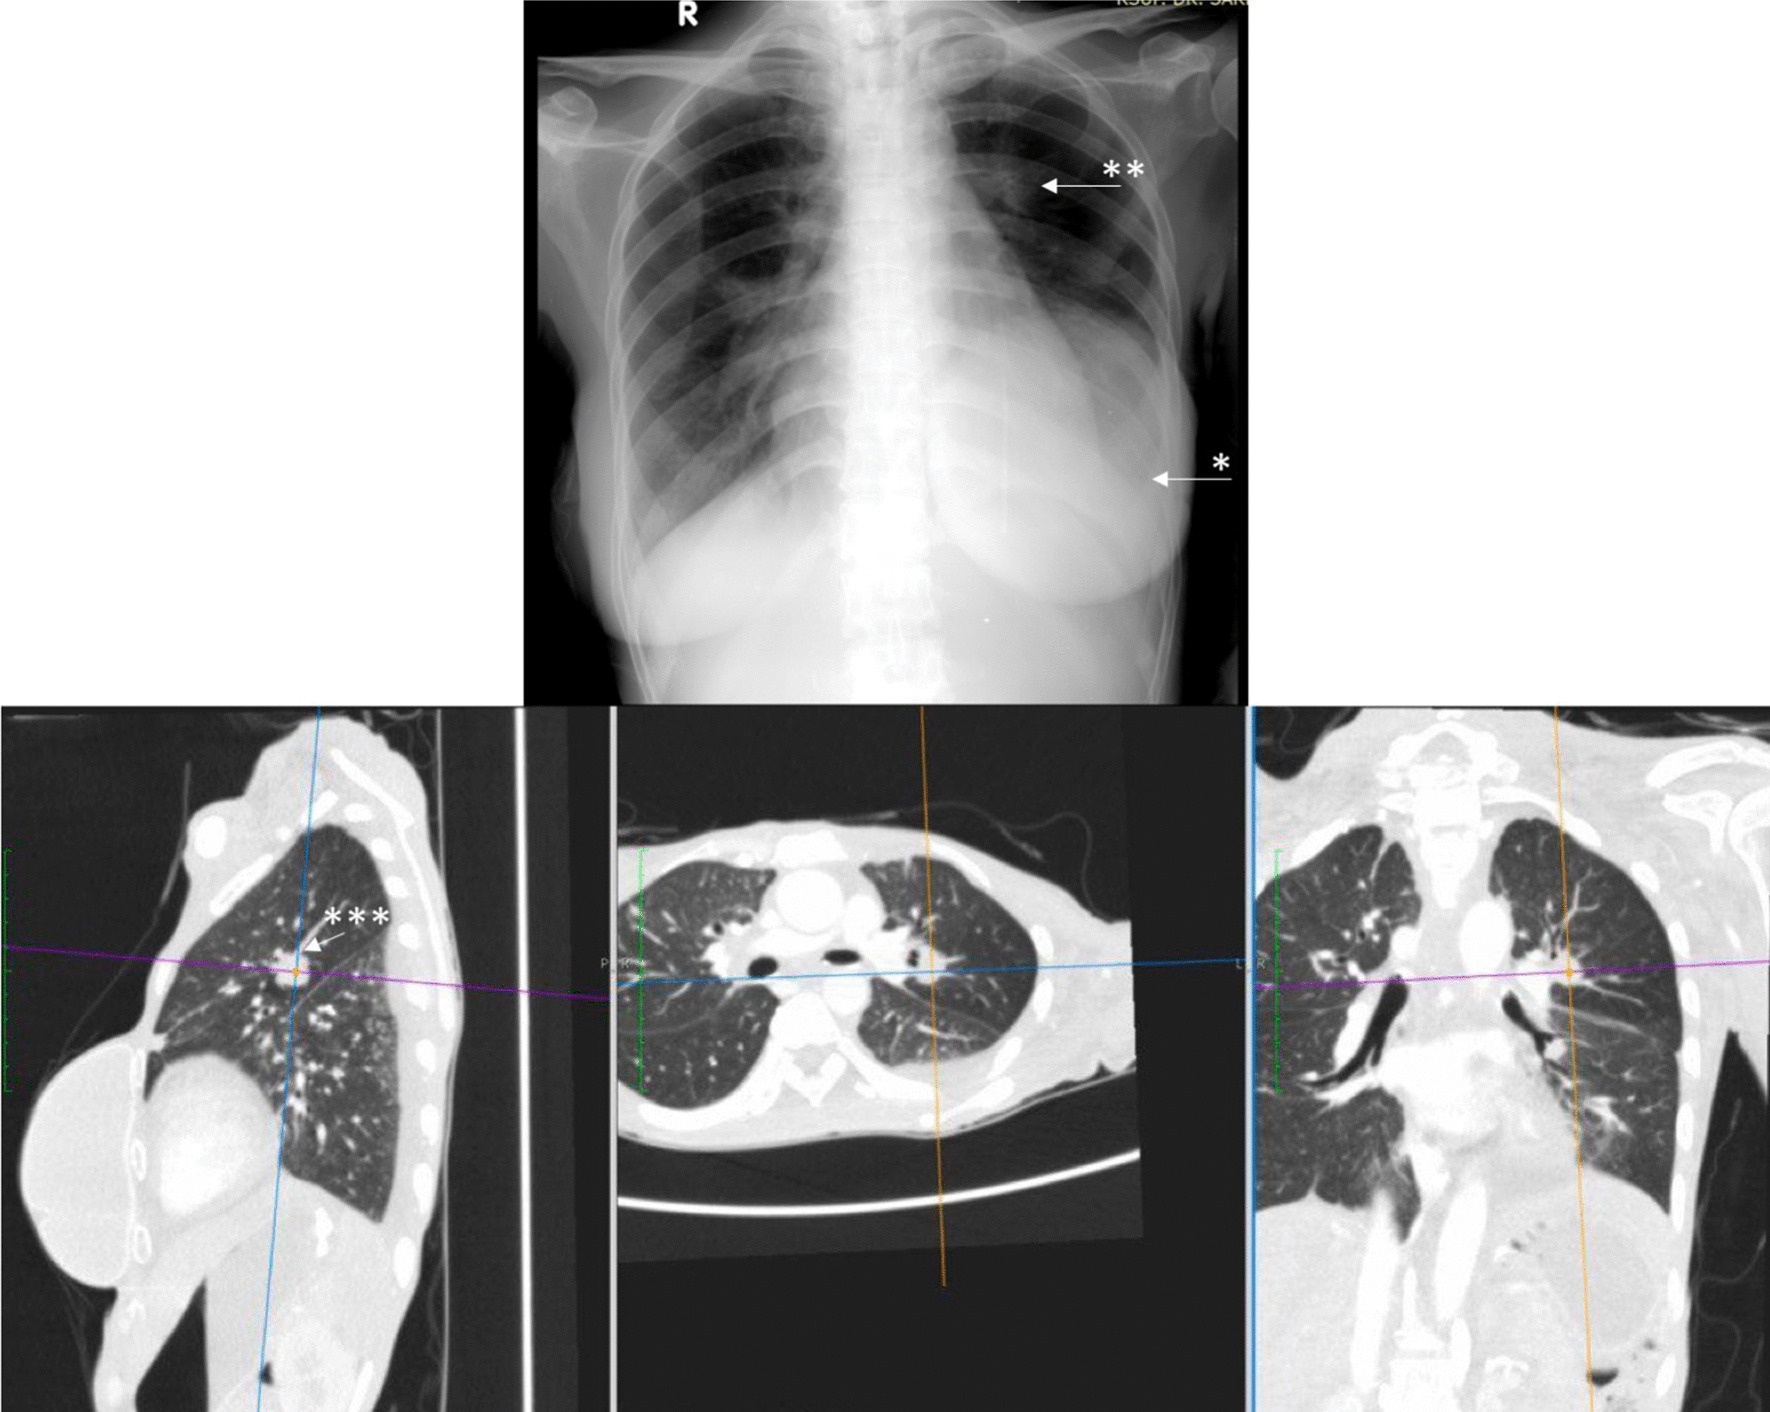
Thoracic X-ray and computed tomography (CT) scan indicate disease spreading into the left pleura and lung. Plain X-ray shows left pleural effusion (*) and nodular lung lesion () that were confirmed with CT scan (*)
